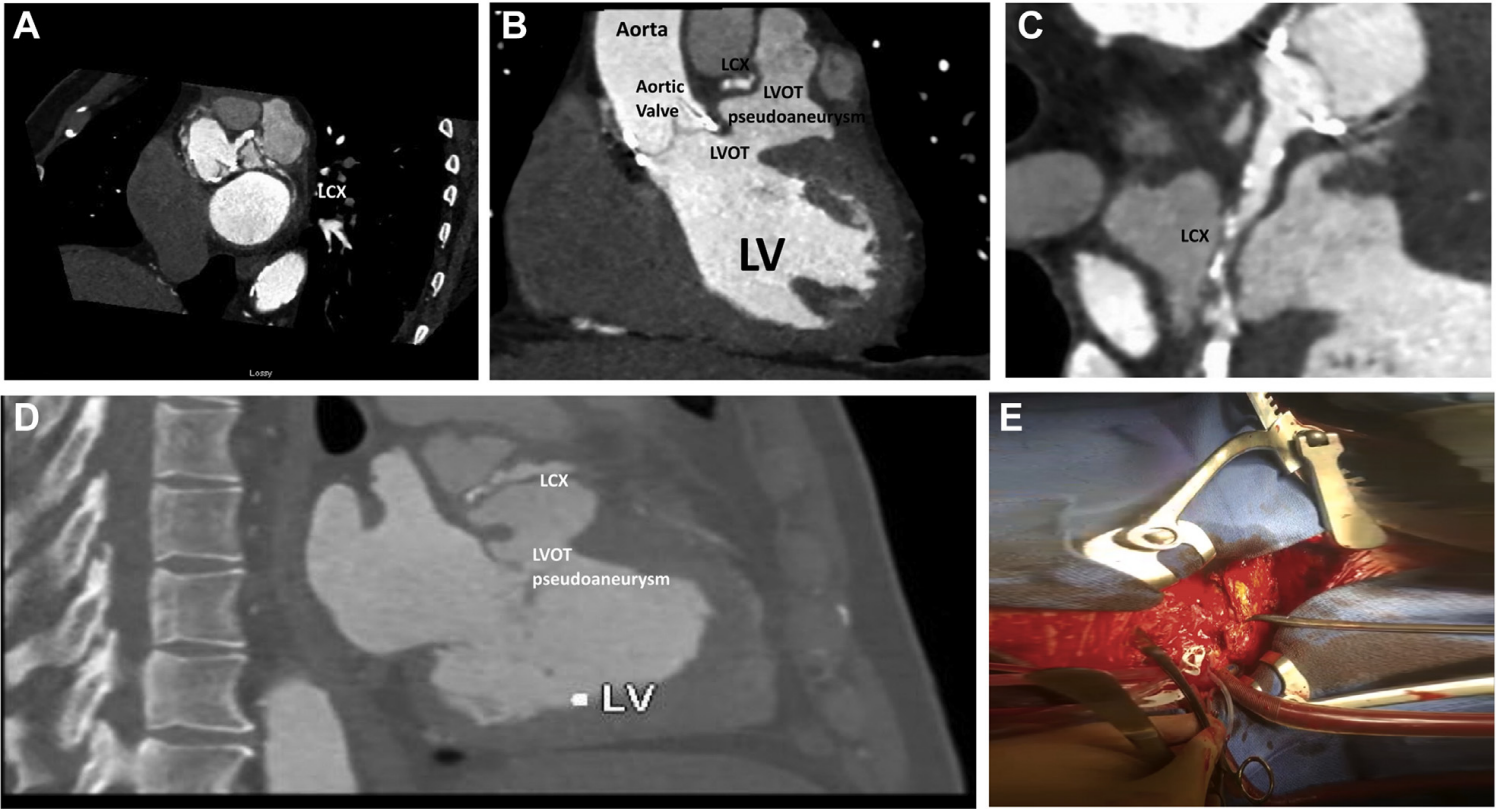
CT and Intraoperative Images of LVOT Pseudoaneurysm (A to D) Computed tomography (CT) of aorta/coronary showing left circumflex (LCX) compression by left ventricular outflow tract (LVOT) pseudoaneurysm. (E) Bleeding into free space from LVOT pseudoaneurysm.
